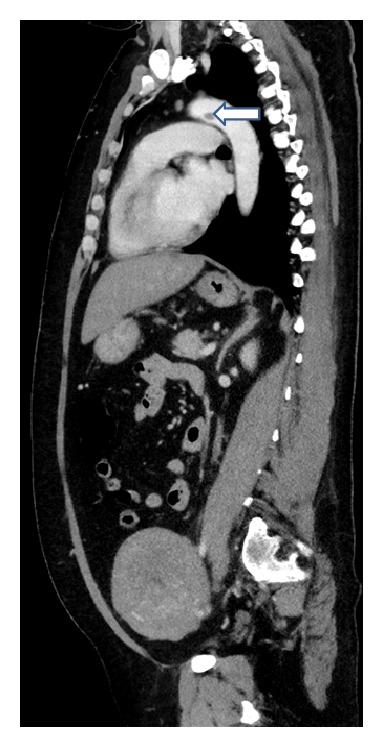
Chest computed tomography demonstrates thrombus (arrow) in the transverse aorta (sagittal view).
